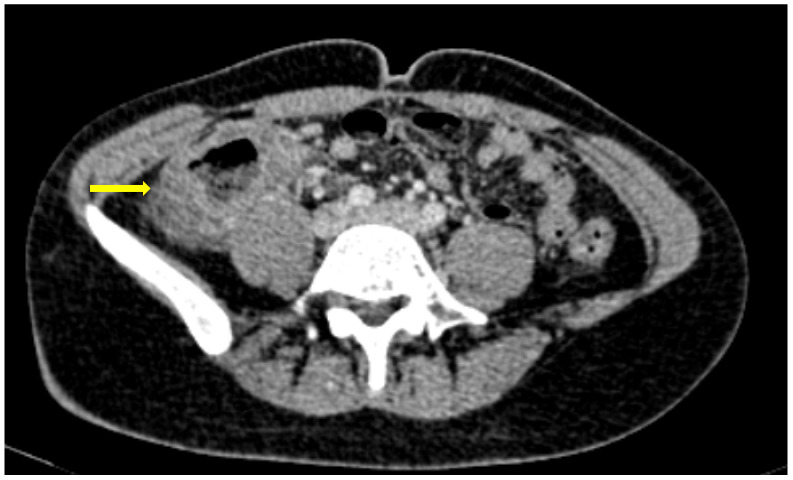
A 48-year-old female patient with COVID-19 pneumonia and no other comorbidities. CT scan depicted a thickened cecum with proximal fat stranding (yellow arrow).
